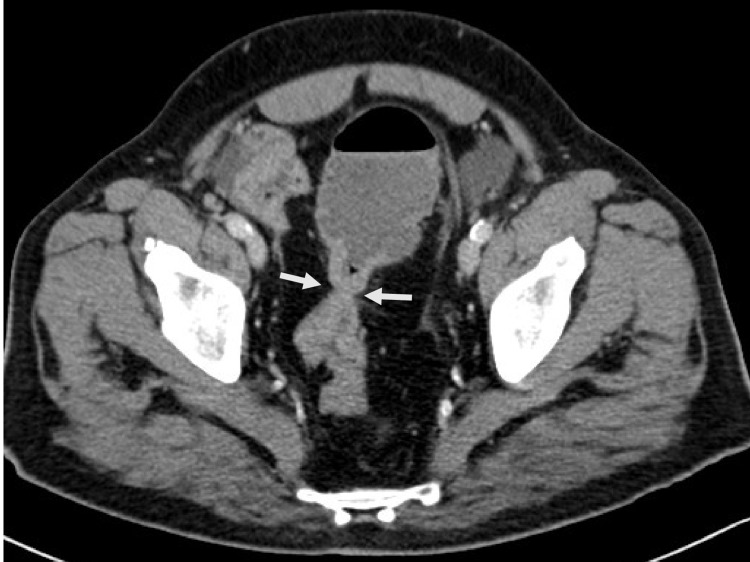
CT scan in the axial plane pelvic cut showing apple-core appearance (arrows) of the rectosigmoid mass lesion keeping with malignancy.
